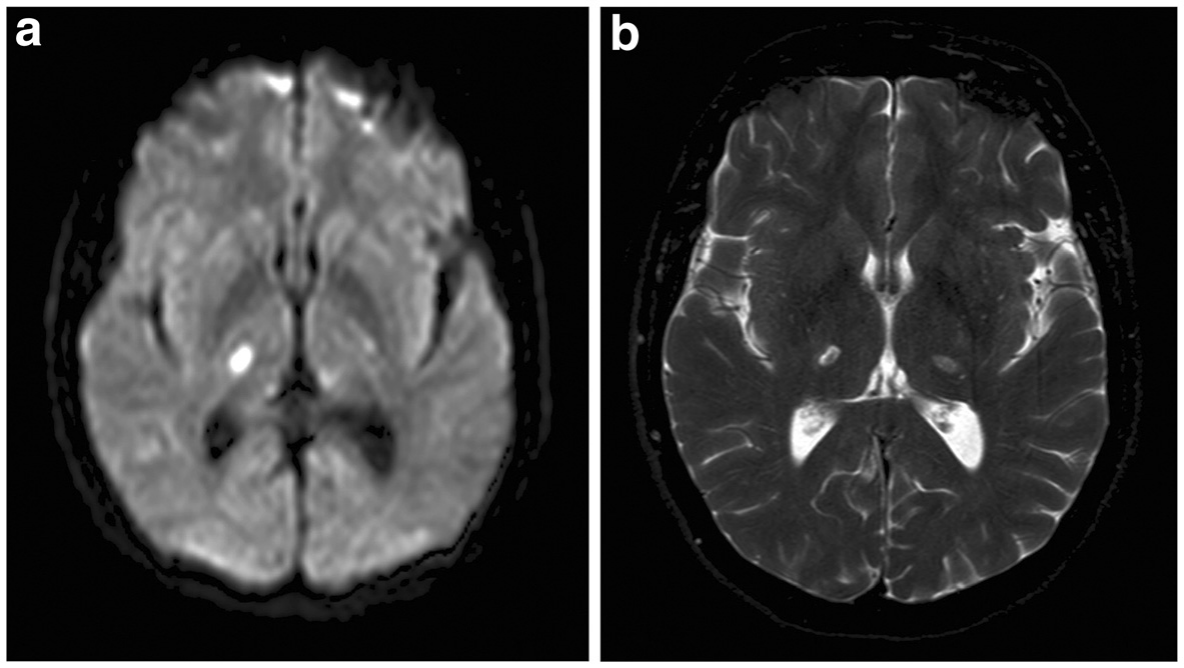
(a) Diffusion-weighted magnetic resonance imaging 6 days after an acute infarction in the right inferolateral artery territory. (b) T2-weighted magnetic resonance image a fortnight later shows bilateral infarctions.
